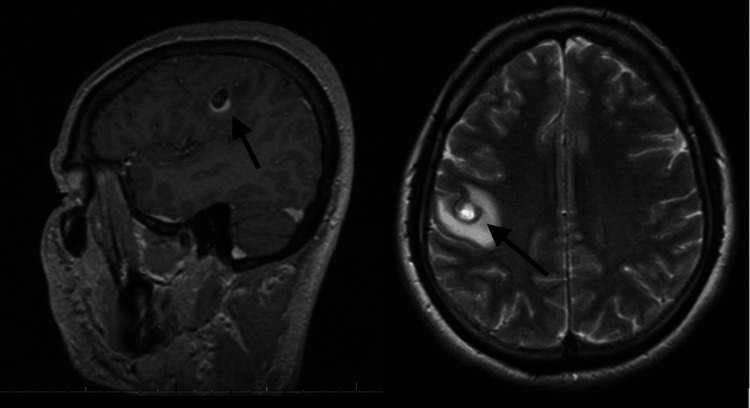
MRI with contrast displaying the ring-enhancing solitary brain lesion, as indicated by the black arrow in both the sagittal and axial cuts
